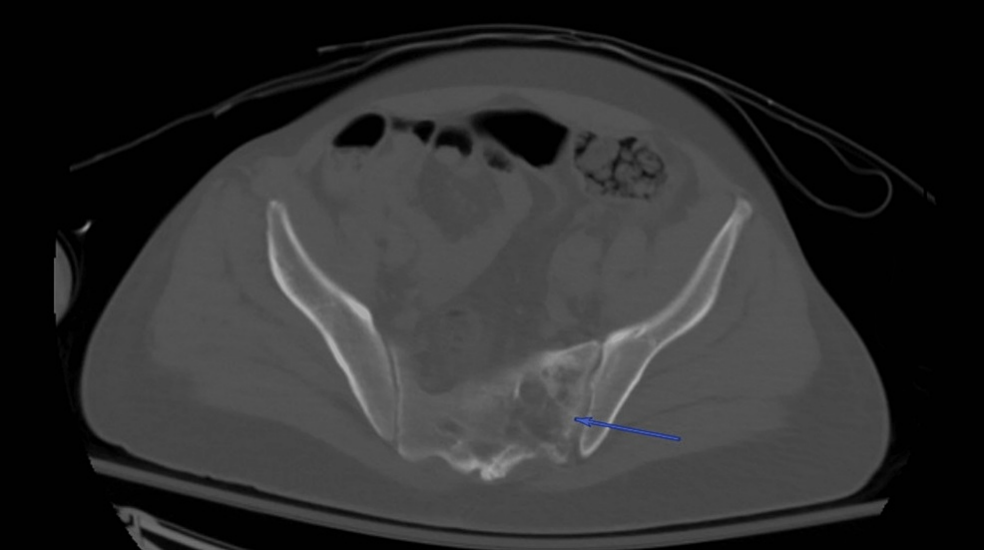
Axial bone window CT scan of the pelvis without intravenous contrast revealing lytic sacral lesions (blue arrow) CT: computed tomography
